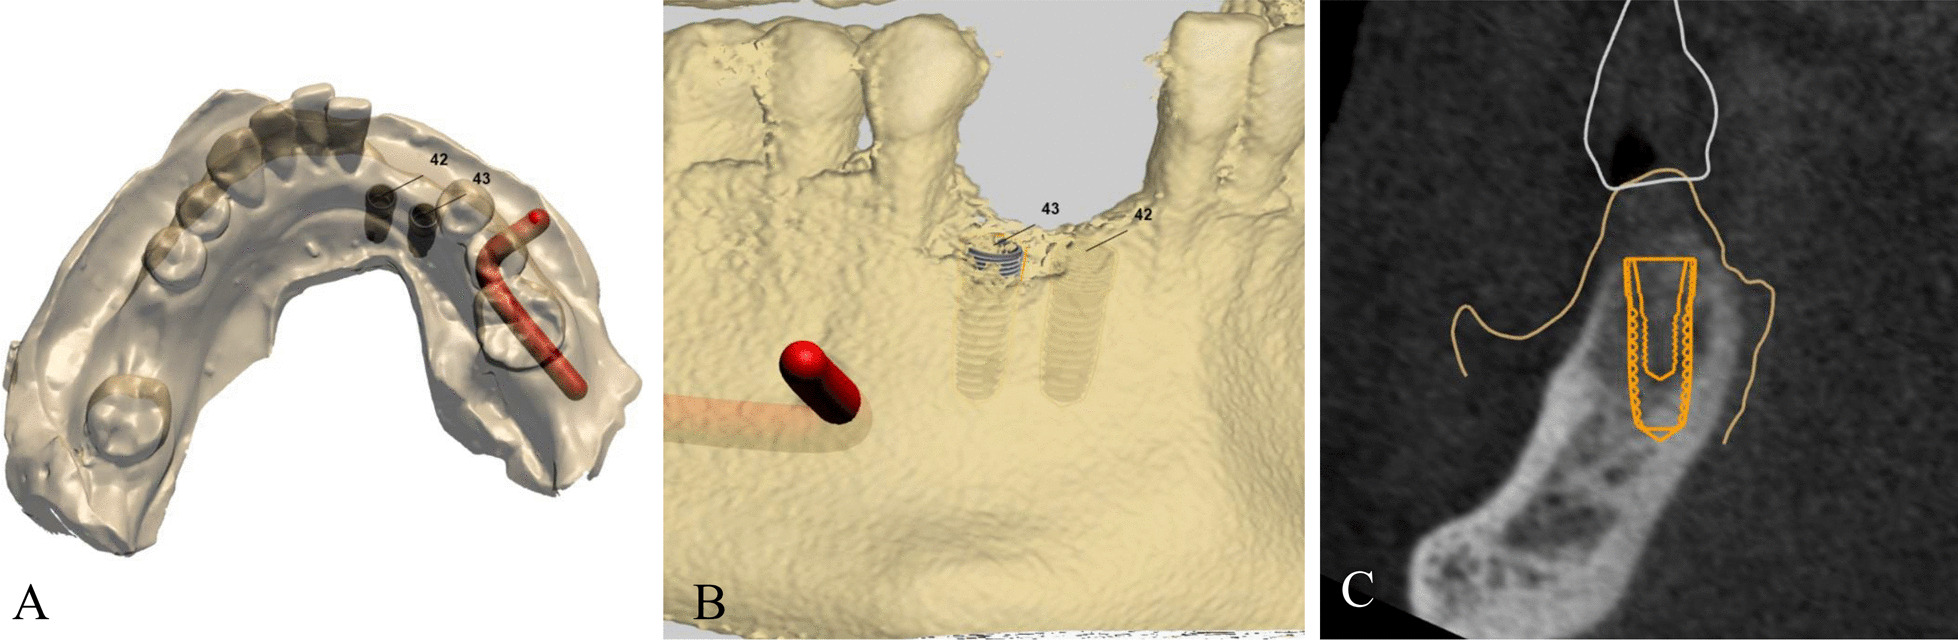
Superimposing the cone beam computed tomography and the intraoral scan. A, B Three-dimensional virtual images of the surgical site. C Virtual implant position planning with the relation of the implant to the surrounding bone structure
